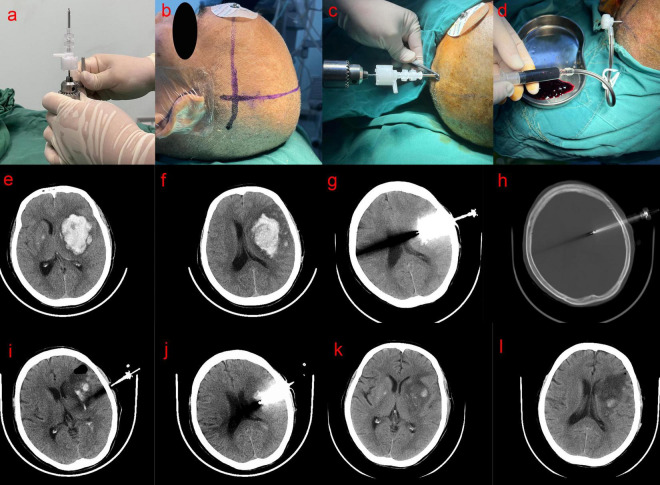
(a) Mounting of the YL-1 hematoma crushing needle on the drill; (b) positioning puncture point; (c) perpendicular drilling (d) aspirated; (e,f) preoperative head CT scan showed the hematoma volume about 45 mL; (g,h) CT scan on the first day after operation showed that the YL-1 puncture needle penetrated the skull into the hematoma cavity; (i,j) CT scan on the 4th day after operation showed that hematoma was almost evacuated; (k,l) 10 days after the operation, head CT showed that the hematoma was completely evacuated.
